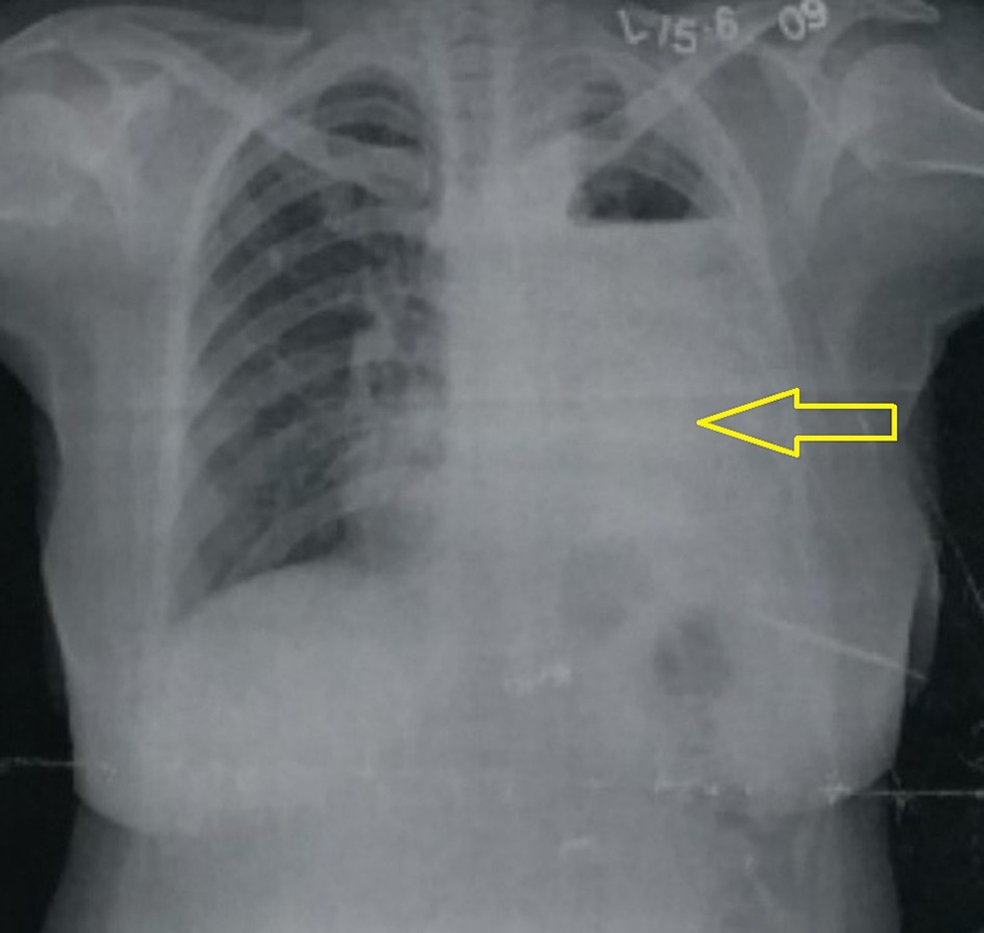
Chest X-ray demonstrating left pneumonectomy status with right functional lung.
